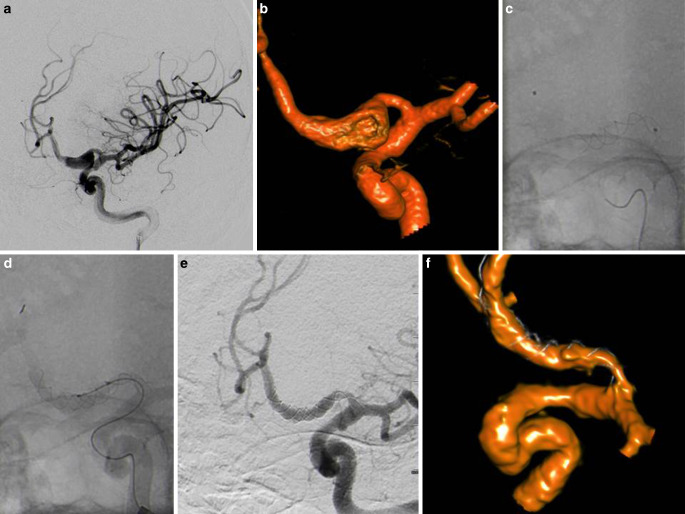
Incidental dissecting aneurysm of the left A1-segment in DSA and FD-CT with intraarterial contrast application (a, b). Placement of two overlapping Leo+ Baby (Balt, Montmorency; 2.5 × 25 and 2.5 × 18 mm; c, d). Follow-up after 5.6 months shows complete occlusion in DSA and FD-CT with intraarterial contrast application (f). FD-CT is of limited quality due to movement artifacts
